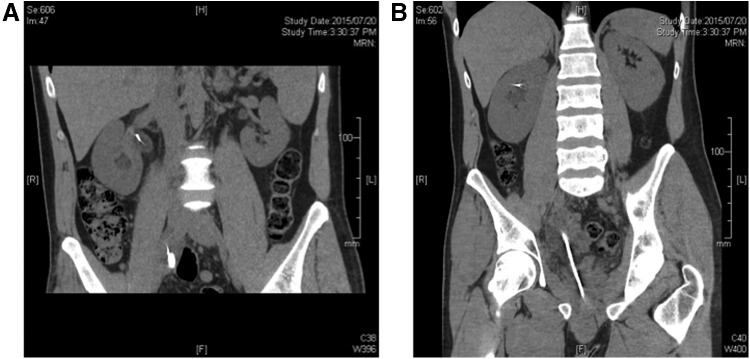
(A, B) CT demonstrating the wider proximal tip of the scope and the rippling appearance of the shaft.
